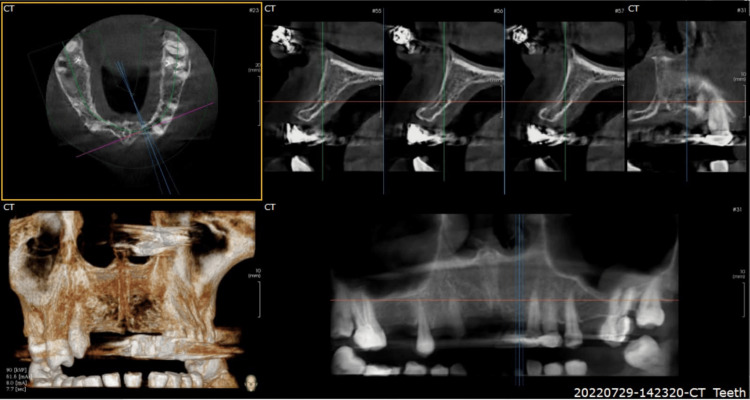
Pre-operative cone-beam computed tomography (Case 1)
